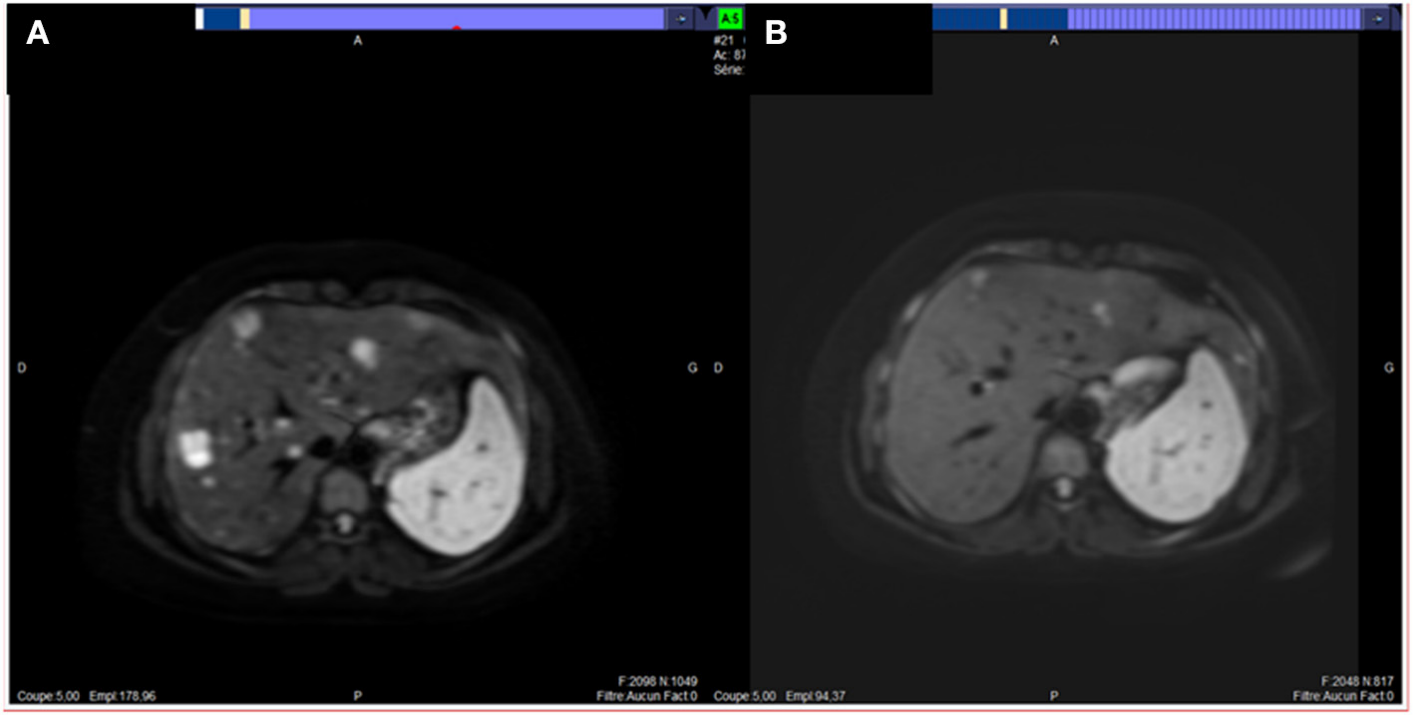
MRI images of liver metastases of our second case of pancreatoblastoma (patient # 2) before (A) and after four cycles (B) of FOLFIRINOX regimen: partial response.
